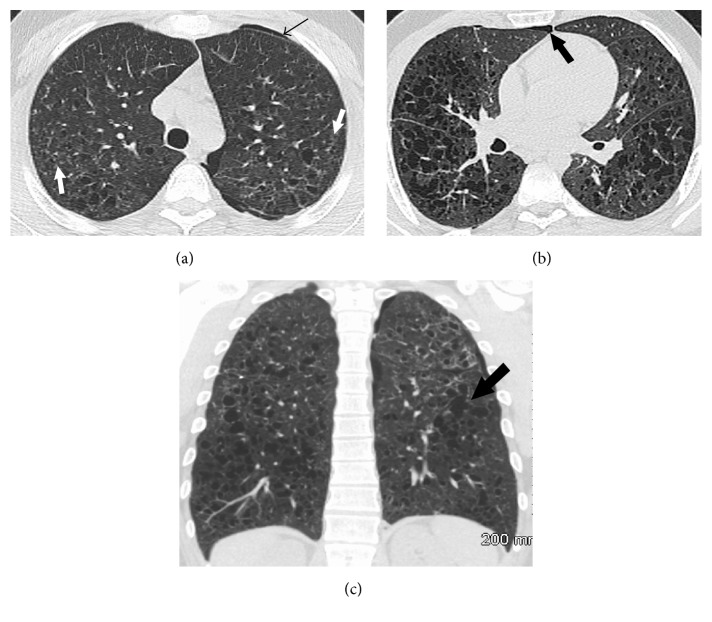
(a) Axial CT scan of the chest with lung window settings at the level of the upper trachea demonstrates multiple bilateral thin-walled cysts and a few ill-defined centrilobular nodules (white arrows). Note a small left pneumothorax (thin black arrow). (b) Axial CT scan of the chest with lung window settings demonstrates bilateral thin-walled cysts, some with bizarre shapes such as in the left lower lobe. Note a small residual right-sided pneumothorax (arrow). (c) Coronal CT reformats with lung window settings demonstrate bilateral lung cysts, some with bizarre shapes (arrow) involving mainly the mid and upper lung zones with relative sparing of the costophrenic angles.
